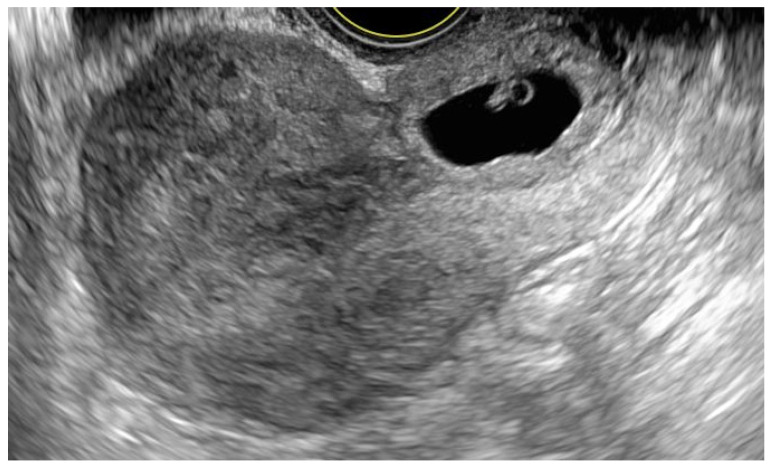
Two-dimensional transvaginal ultrasound image in sagittal plane demonstrating a gestational sac located within cervical canal.
